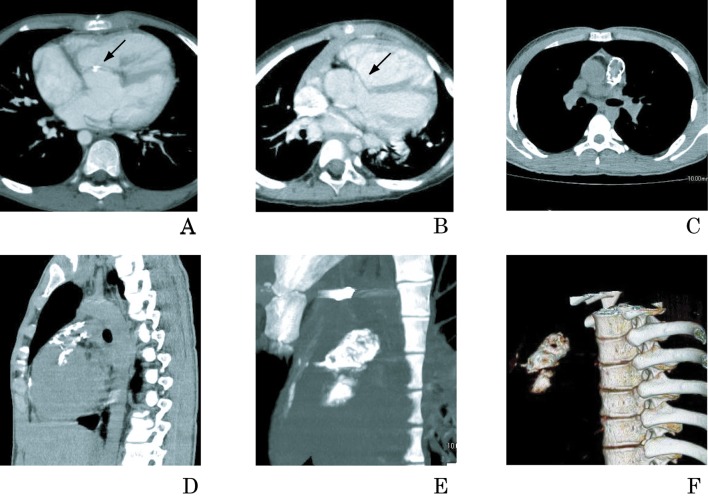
Multidetector-row computed tomographic views of PTFE grafts used for VSD patches and RVOT prostheses. (A) Five-year-old boy with tetralogy of Fallot (TOF) who underwent complete repair with placement of a VSD patch. The axial view reveals spot calcification (arrow) on the VSD PTFE patch. (B) Twelve year-old boy with double outlet right ventricle (DORV) who underwent complete repair with placement of a VSD patch. Multiplanar reconstruction images show VSD patch (arrow) in the axial slice. No calcification is detected. Twenty-year-old man with TOF who underwent complete repair with placement of PTFE graft in RVOT reconstruction. Multiplanar reconstruction images show severe calcification of RVOT in axial (C) and sagittal (D) views. Maximum intensity projection (E) and volume-rendered reconstruction (F) provide spatial and 3D aspects. *Reproduced with permission from American Heart Journal 2007; 153: 806.e1-8, Mosby, Inc.*
